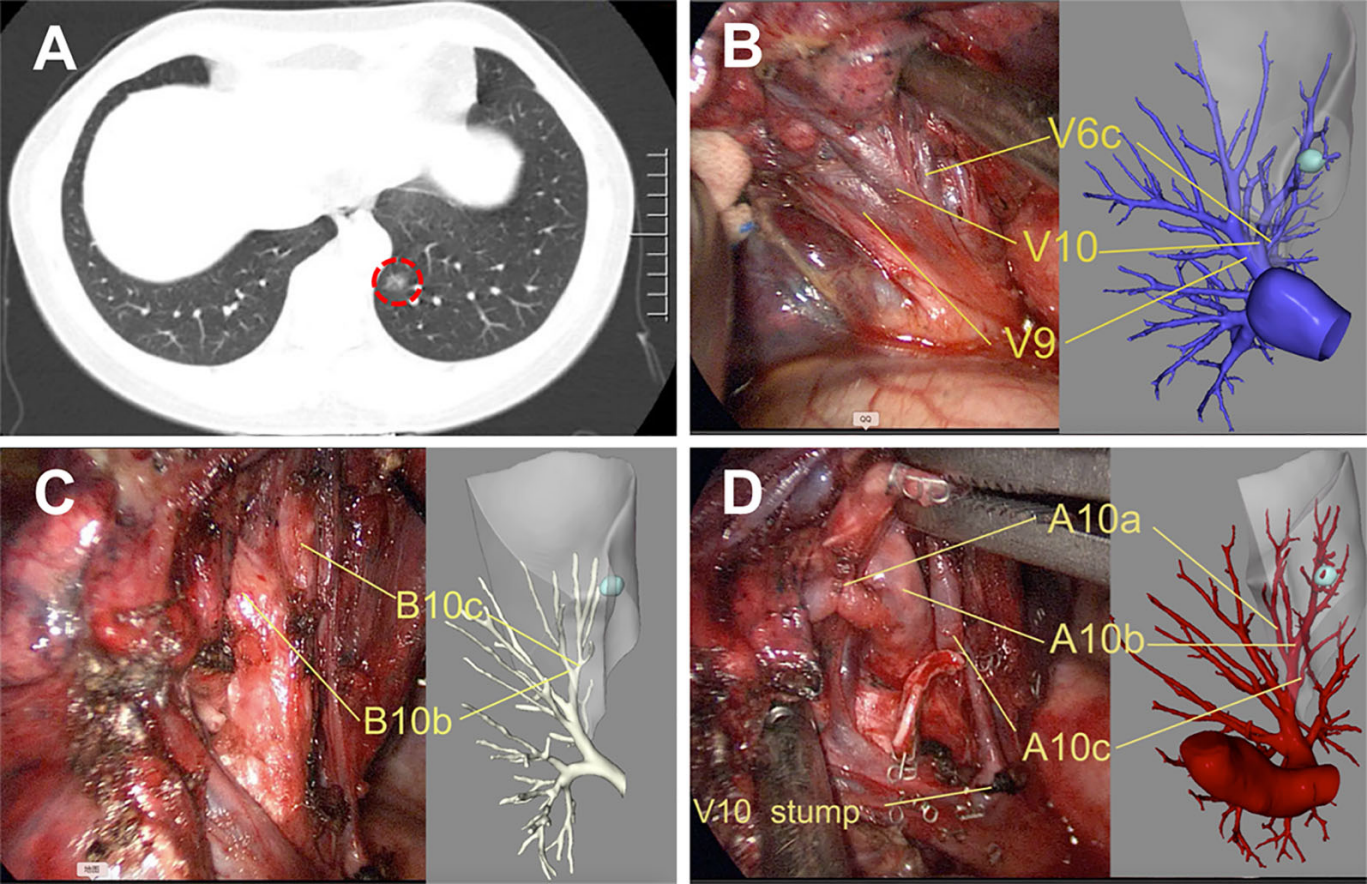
Precise left S^10^ segmentectomy according to the real-time 3D reconstruction guidance. (A) CT scan showed a GGOs lesion in the left S^10^; Branches pulmonary vein (B), bronchus (C), and pulmonary artery (D) of the target segment were confirmed by real-time guidance of 3D reconstruction. 3D, three-dimensional.
